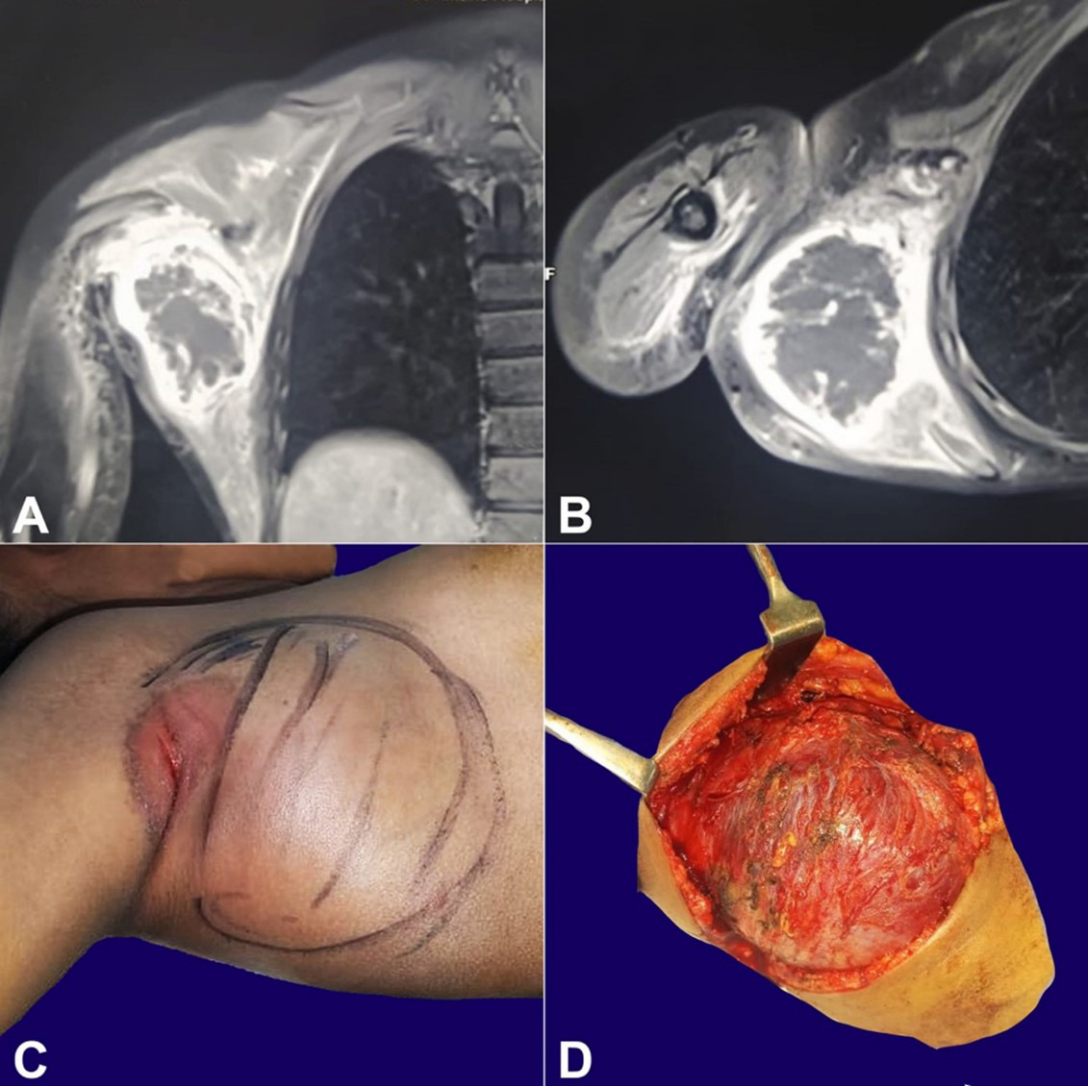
A and B – computed tomography of the shoulder; A – coronal plane; and B ­– axilla plane, image delineating the lesion with curvilinear peripheral ossification and central lucency; C and D – Pre-operative and intra-operative images of the lesion.
